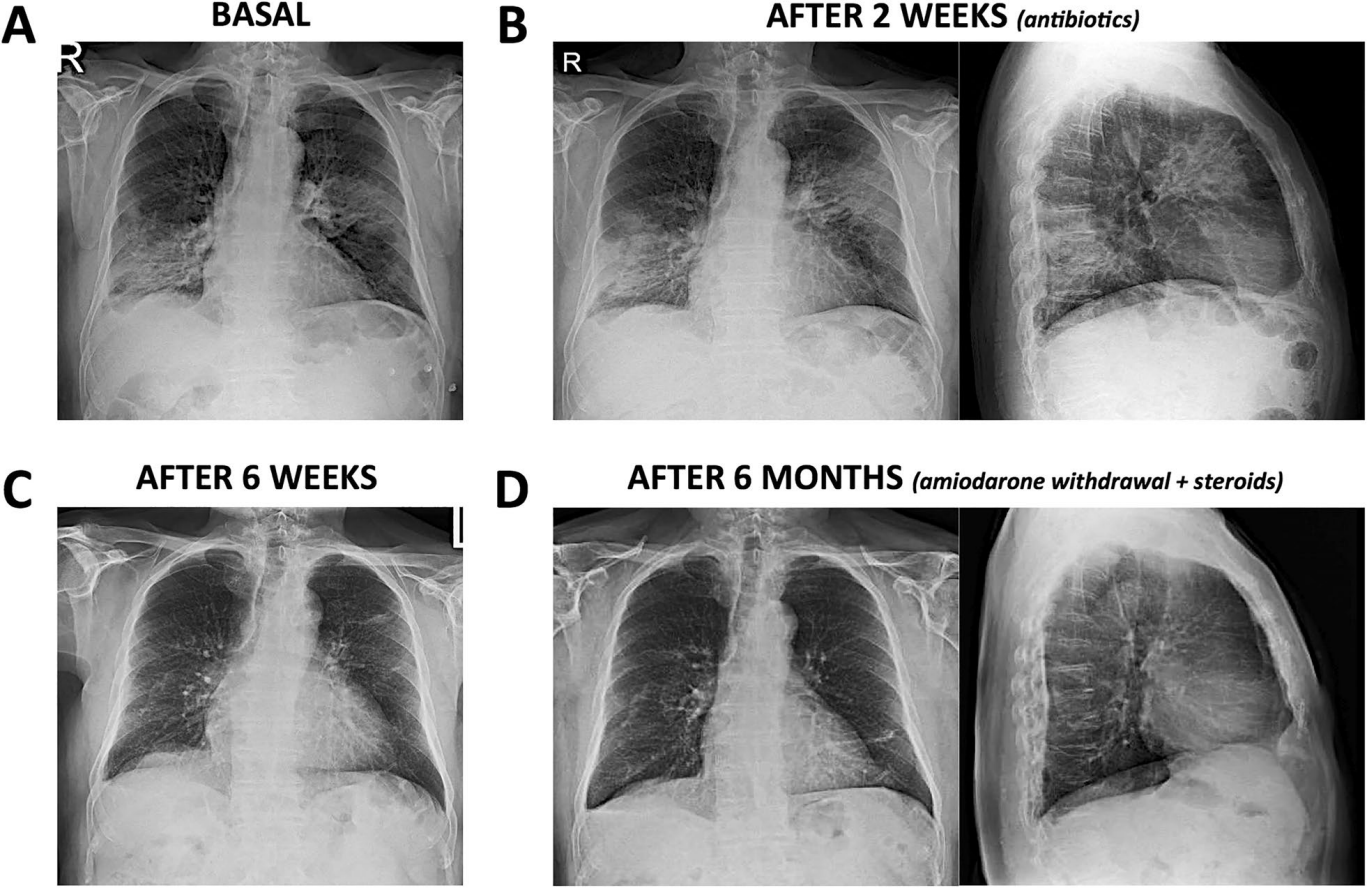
Chest X-rays. A, B Interstitial-alveolar opacities involving the posterior segment of the right lower lobe and the anterior segment of the left upper lobe; left hilar enlargement; and right basal pleural effusion. Baseline imaging was substantially unchanged after antibiotic therapy. C, D Progressive clearing and resolution of parenchymal opacities, with only right pleural thickening and costophrenic angle obliteration remaining after amiodarone discontinuation and steroid therapy
